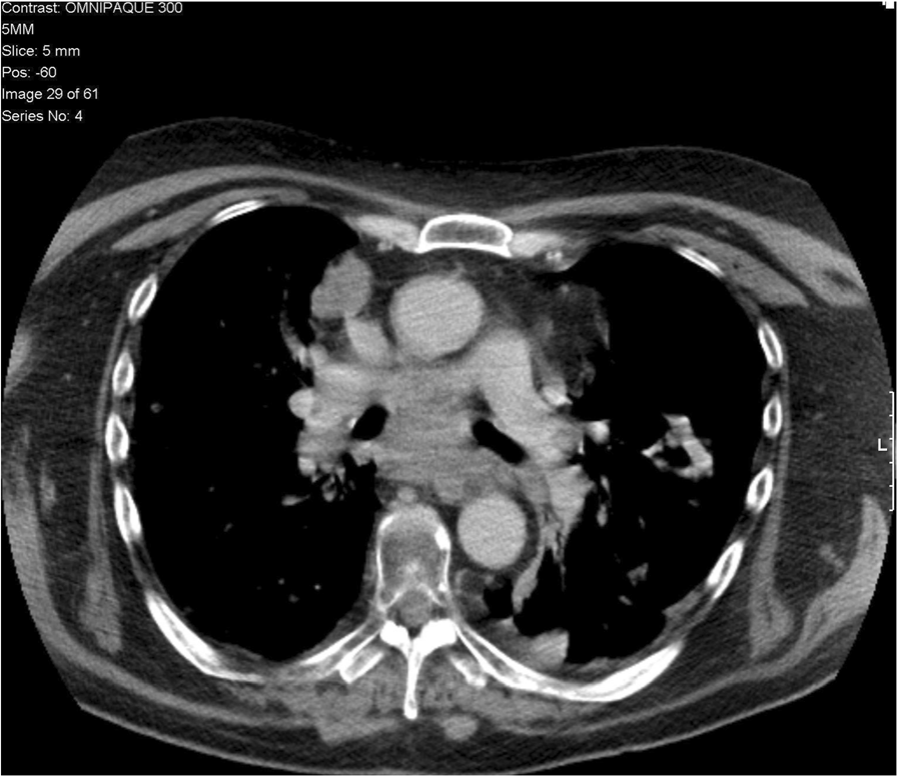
Chest CT showing disease response. Staging CT scan after 4 weeks of crizotinib treatment showing disease response with improvement in mediastinal nodal disease, lung metastases, and re-canalization of the left main bronchus.
